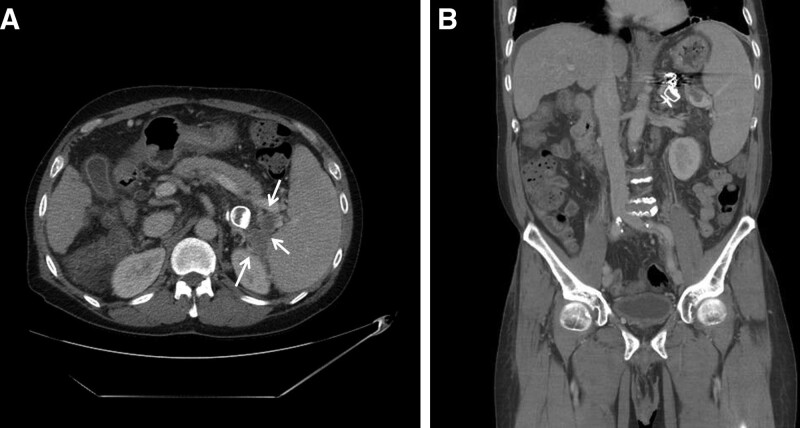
CT axial (A) and coronal (B) images performed 7 days later showed vascular plugs and coils in the splenorenal shunt, and the perisplenic collaterals was filled with thrombus. CT = computed tomography.
